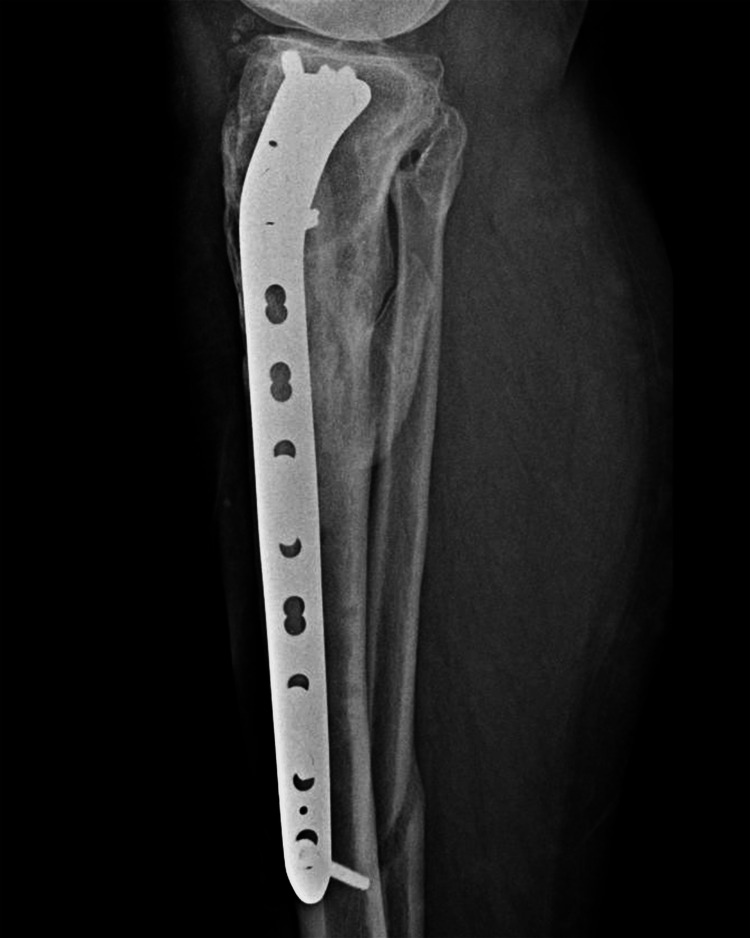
Lateral X-ray of the right leg taken at a two-year postoperative follow-up, showing the bone union and visible fibula shaft autograft in the proximal tibia medullary canal
